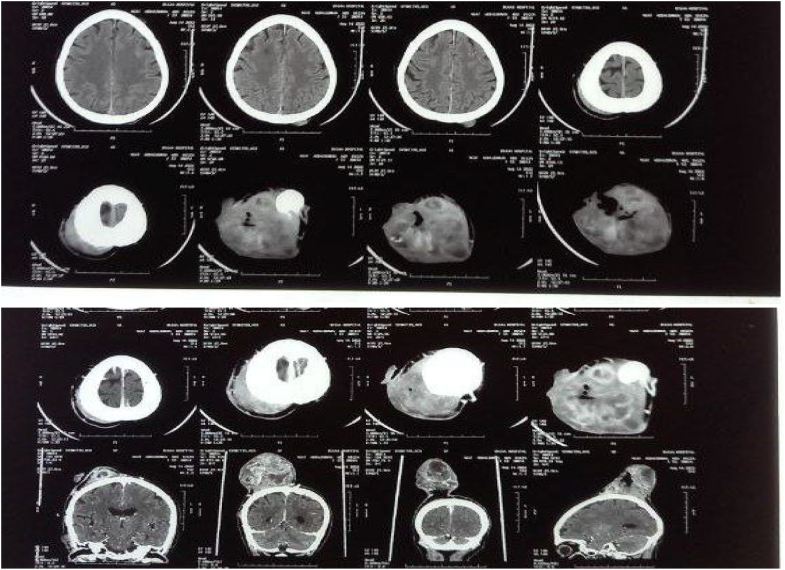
CT scan show will define heterogeneous subcutaneous masses with no evidence of underlying bone destruction, invasion and intracranial lesion or invasion.
